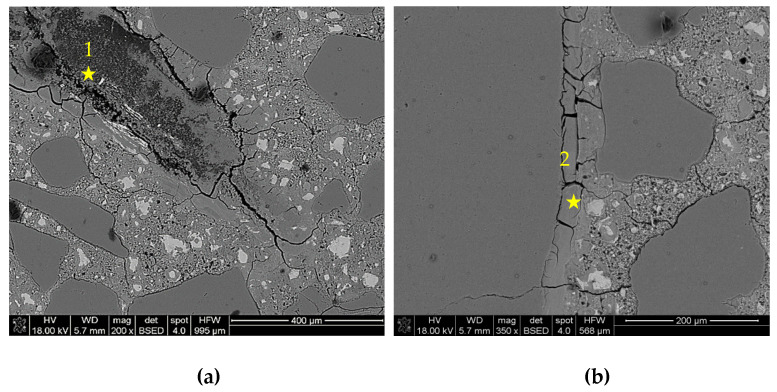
Microstructure images of control mortars: (a) gel in reactive aggregate; (b) gel located in ITZ. X-ray microanalysis at location 1 – 2 (see Table 4).
